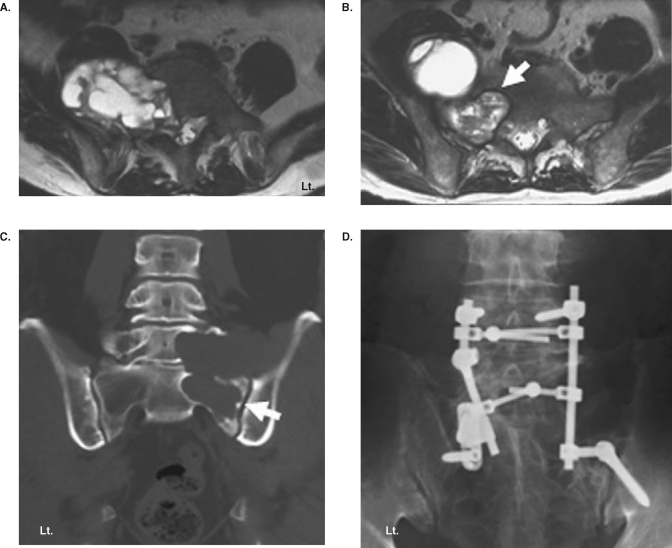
Case 5. Axial images of T2-weighted MRI at L5 level (A) and S1 level (B) showing bony destruction of the vertebral body and a tumor involving the intraosseous area of S1 (white arrow) and presacral region. Frontal reconstruction of CT (C) showing obvious bony destruction of the L5 body, sacrum, and sacroiliac joint (white arrow). Partial tumor excision was done using the posterior approach, and lumbopelvic fixation and bone grafting were performed (D).
